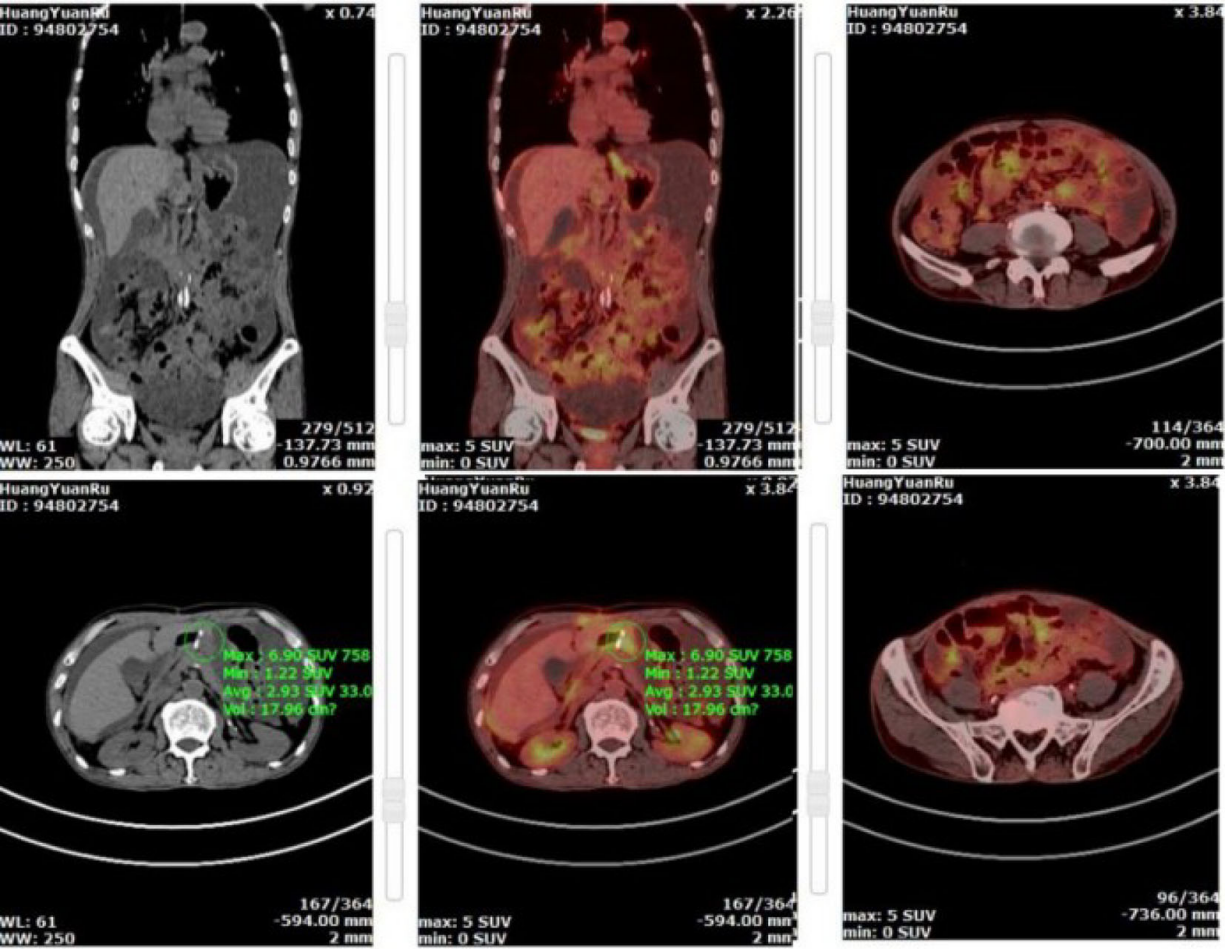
Pet-CT showed tumor recurrence at anastomosis with multiple peritoneal metastases.
